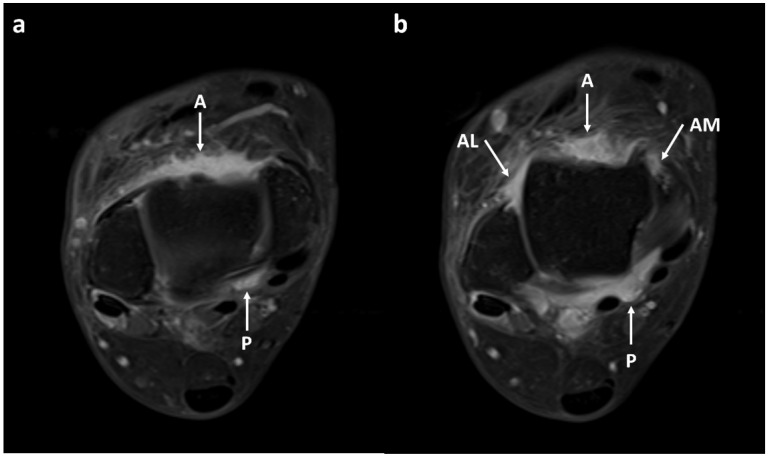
Axial contrast-enhanced, T1-weighted images showing synovial enhancement. The ankle joint was divided into four compartments: anterior recess (A), anteromedial gutter (AM), anterolateral gutter (AL), and posterior recess (P). (a) The anterior recess (A) was defined as the central portion of the recess between the anterior tibial plafond and the talar dome. The posterior recess (P) was defined as the posteromedial recess formed anteriorly by the medial malleolus and posterior tibiotalar ligament, laterally by the talar dome and posterior process of the talus, and peripherally by the flexor hallucis longus tendon and neurovascular bundle. (b) The anteromedial gutter (AM) was the space formed superficially by the joint capsule, laterally by the talus, medially by the medial malleolus, and inferiorly by the anterior tibiotalar ligament. The anterolateral gutter (AL) was the space formed medially by the tibia, laterally by the fibula, superiorly by the anteroinferior tibiofibular ligament, inferiorly by the calcaneofibular ligament, and anteriorly by the anterior talofibular ligament and joint capsule. Both readers assigned a synovial visibility grade of 4 (fully visible synovium with good tissue contrast) in all joint compartments. Reader 1 assigned a synovial thickness score of 1 (maximum thickness 2–4 mm) in the anteromedial gutter and 2 (maximum thickness ≥4 mm) in the other compartments. Reader 2 assigned a synovial thickness score of 2 in all joint compartments.
